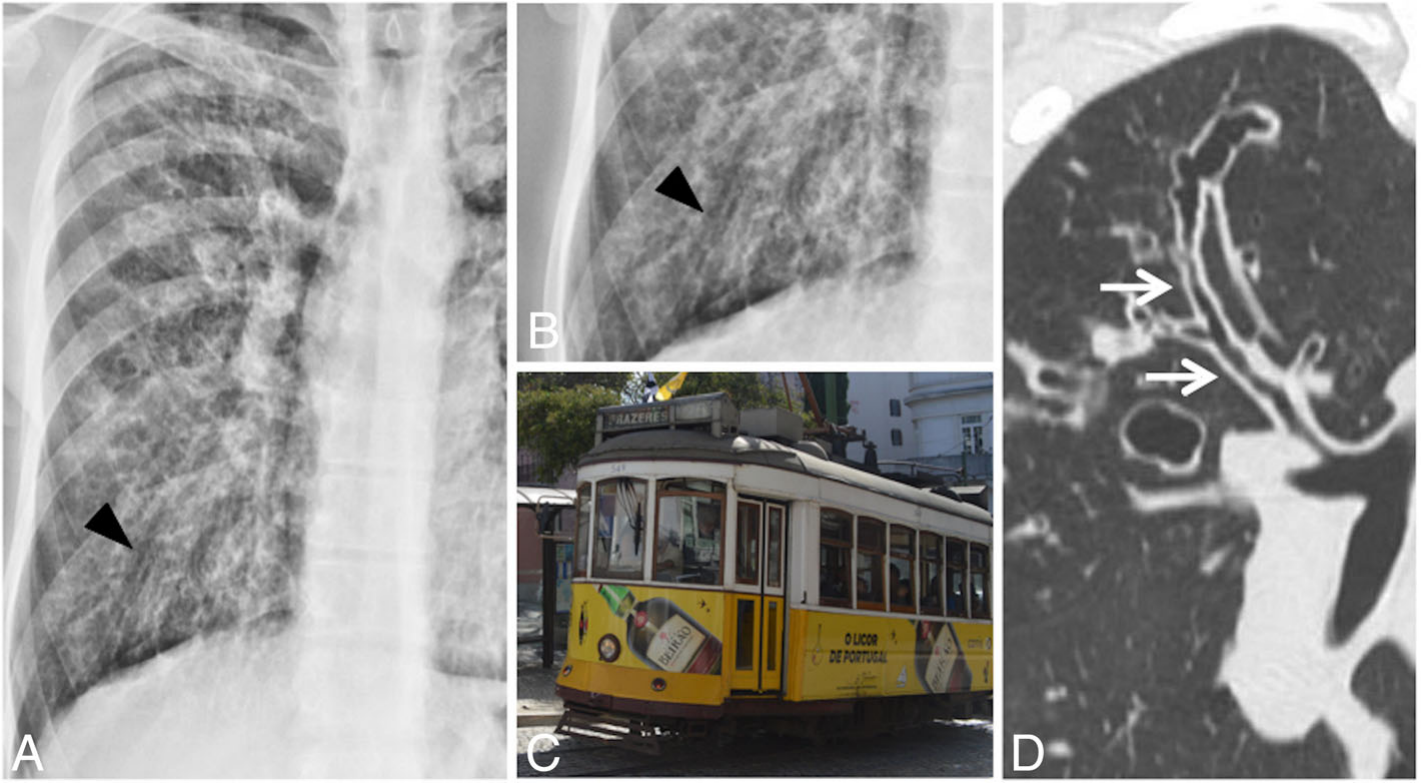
Tram track sign. The tram track sign may be explained by the presence of thickened bronchial branches on radiographs (black arrowheads in a and b), which reproduce a “tram line” appearance clearly shown on c. In d, the same appearance could be also described in a CT of a patient with cystic fibrosis: the coronal CT image shows thickened bronchial branches (cylindrical bronchiectasis)
